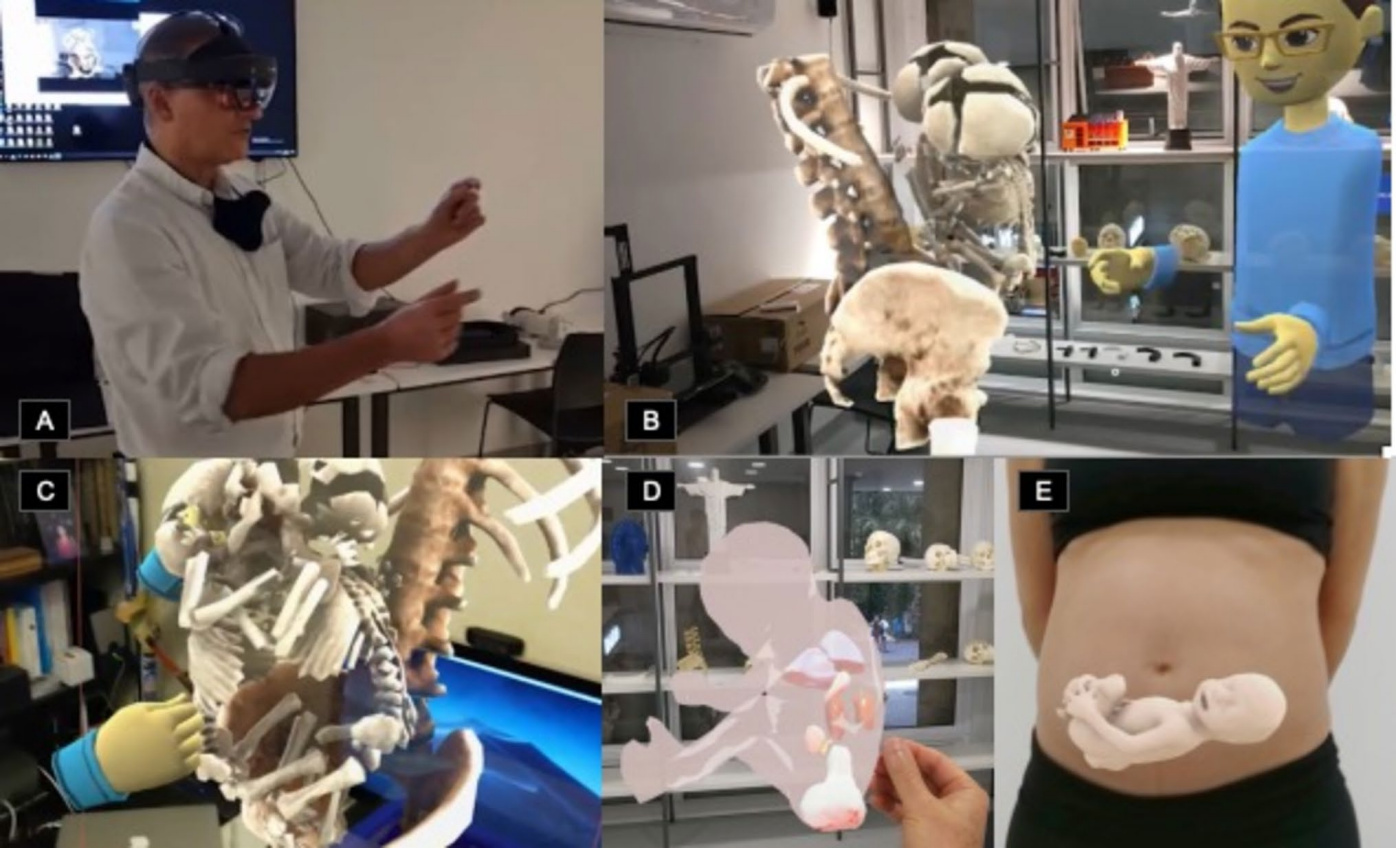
Augmented reality (AR) examples. Images A–C show the potential of AR in planning a conjoined twin surgery in our laboratory. DICOM images from a volumetric abdominal CT were used to process the 3D visualization. Through a head-mounted AR device, (A) it is possible to obtain the 3D images and a perspective view of the conjoined twins with the HMD (B, C). This approach can also be used for other cases such as the prospective evaluation of a fetus with a sacral teratoma superimposed on the real office background (D) or superimposed on the mother’s abdomen (E)
